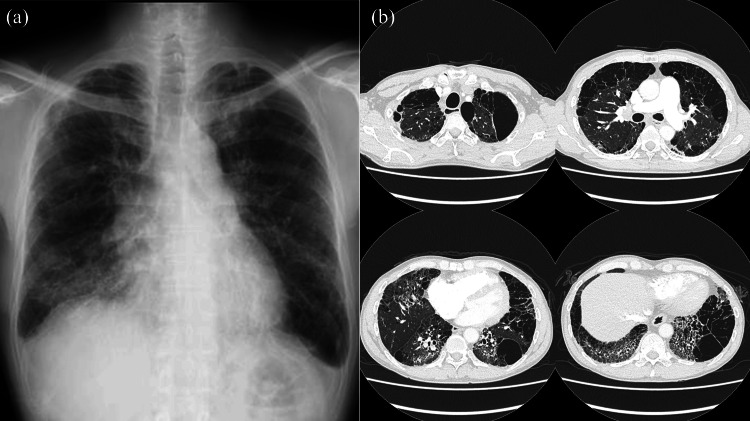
Patient’s chest X-ray (a) and computed tomography (CT) (b) images before bilateral lung transplantation Chest X-ray reveals lower lobe reticulation. High-resolution CT shows upper lobe emphysema and lower lobe honeycombing and traction bronchiectasis.
